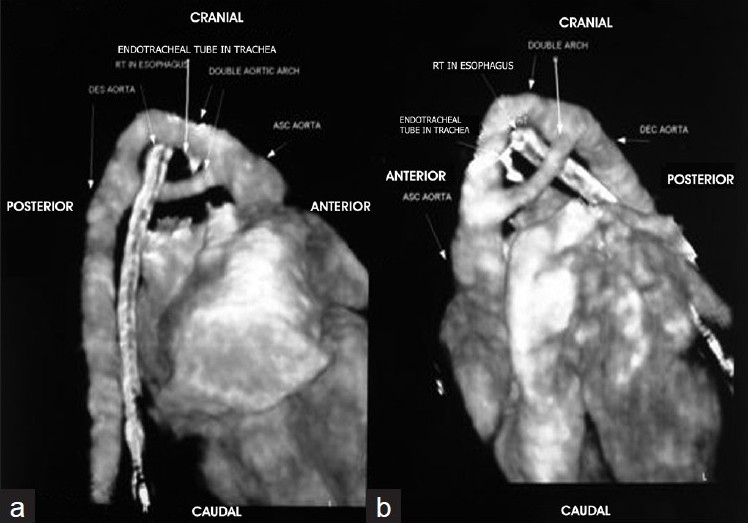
Multidetector computed tomography with 3-dimensional reconstruction images showing double aortic arch encircling the esophagus and trachea with the Ryle’ tube and endotracheal tube *in situ*, respectively
